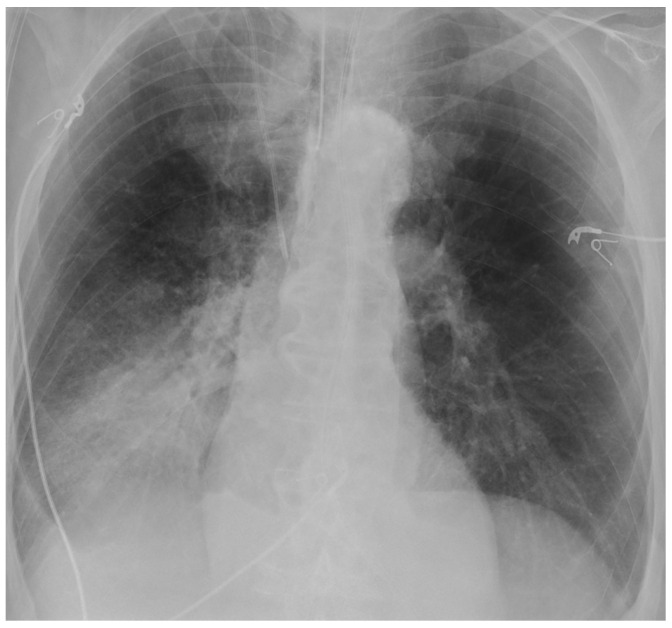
Chest radiograph in an elderly patient with COPD with *Haemophilus influenzae* pneumonia: homogeneous consolidation in the right lower lobe with air bronchograms. Representative image from the anonymized institutional radiology archive of University Hospital Frankfurt.
